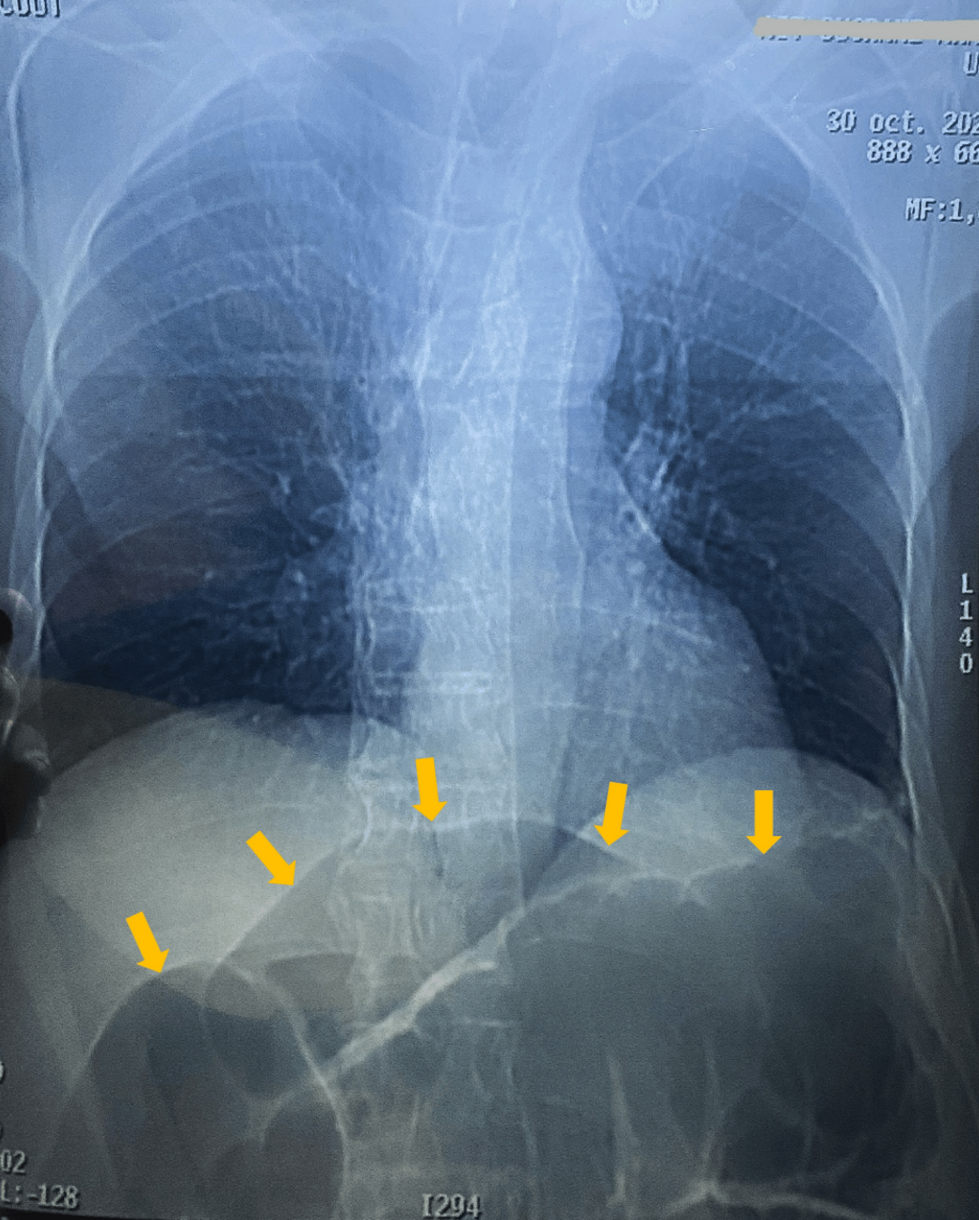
Abdominal X-ray reveals markedly distended, air-filled large bowel loops (arrows), suggestive of intestinal obstruction.
